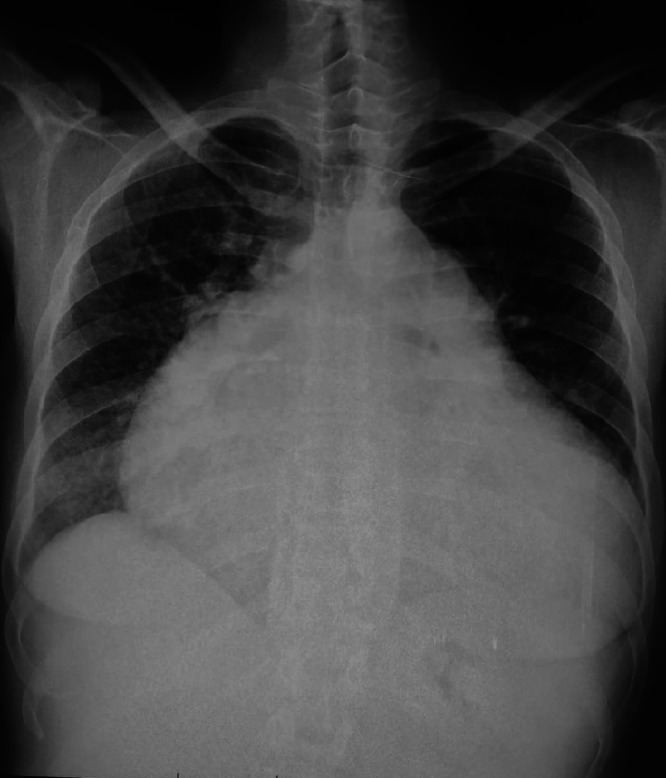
Chest radiography (posteroanterior view) demonstrating cardiomegaly with a prominent right heart border and enlarged main pulmonary artery segment, consistent with chronic pulmonary arterial hypertension.
